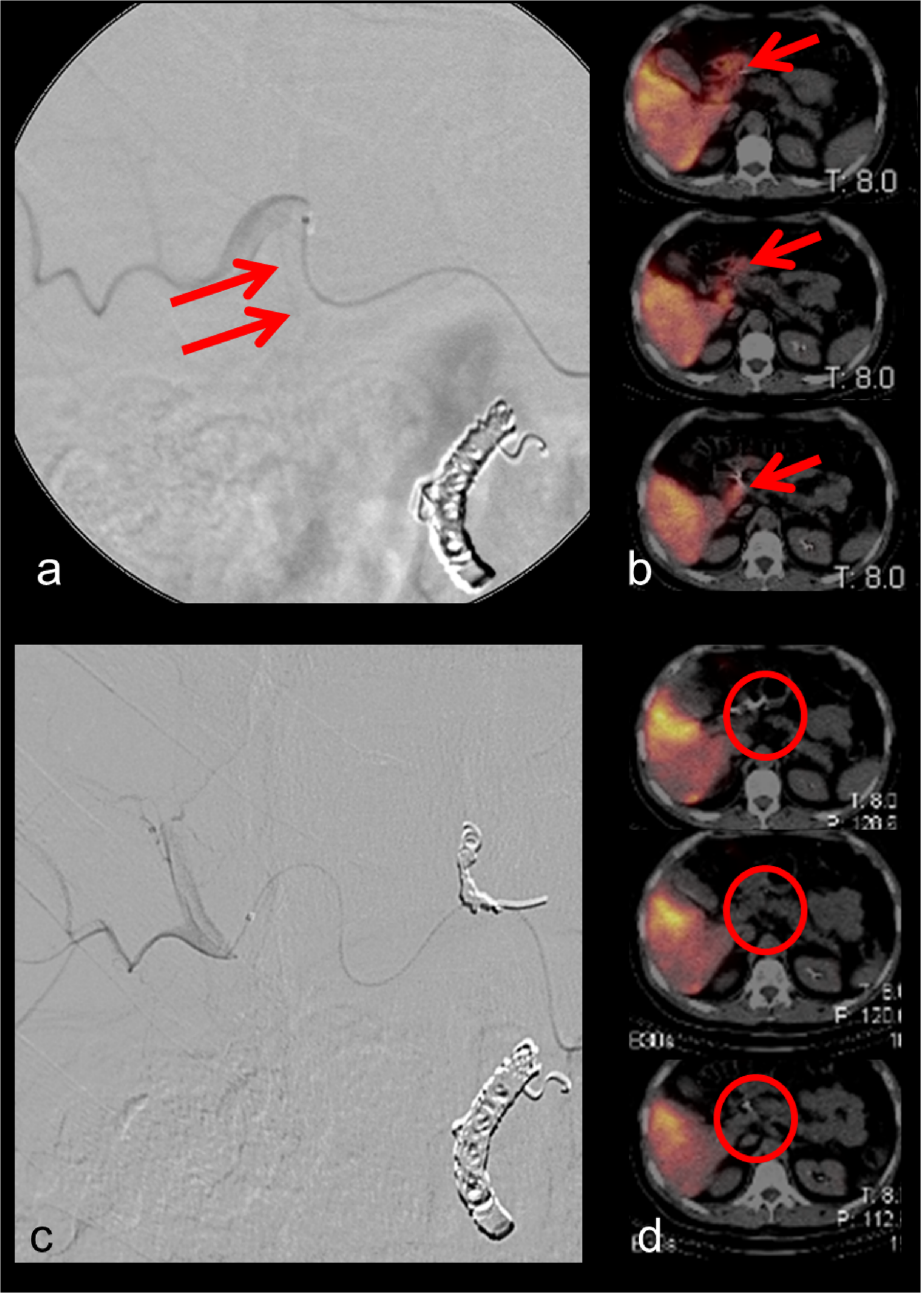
47 year old male patient. During the initial DSA the gastroduodenal artery and right gastric artery were occluded. However, a small branch to the small bowel arising from the right hepatic artery was missed (Fig 3a) resulting in an extrahepatic 99mTc-HSA deposition in the small bowel wall (Fig 3b). During repeated angiography the microcatheter was positioned in a more distal part of the right hepatic artery (Fig 3c). Subsequent SPECT/CT did not reveal any extrahepatic tracer accumulation (Fig 3d).
